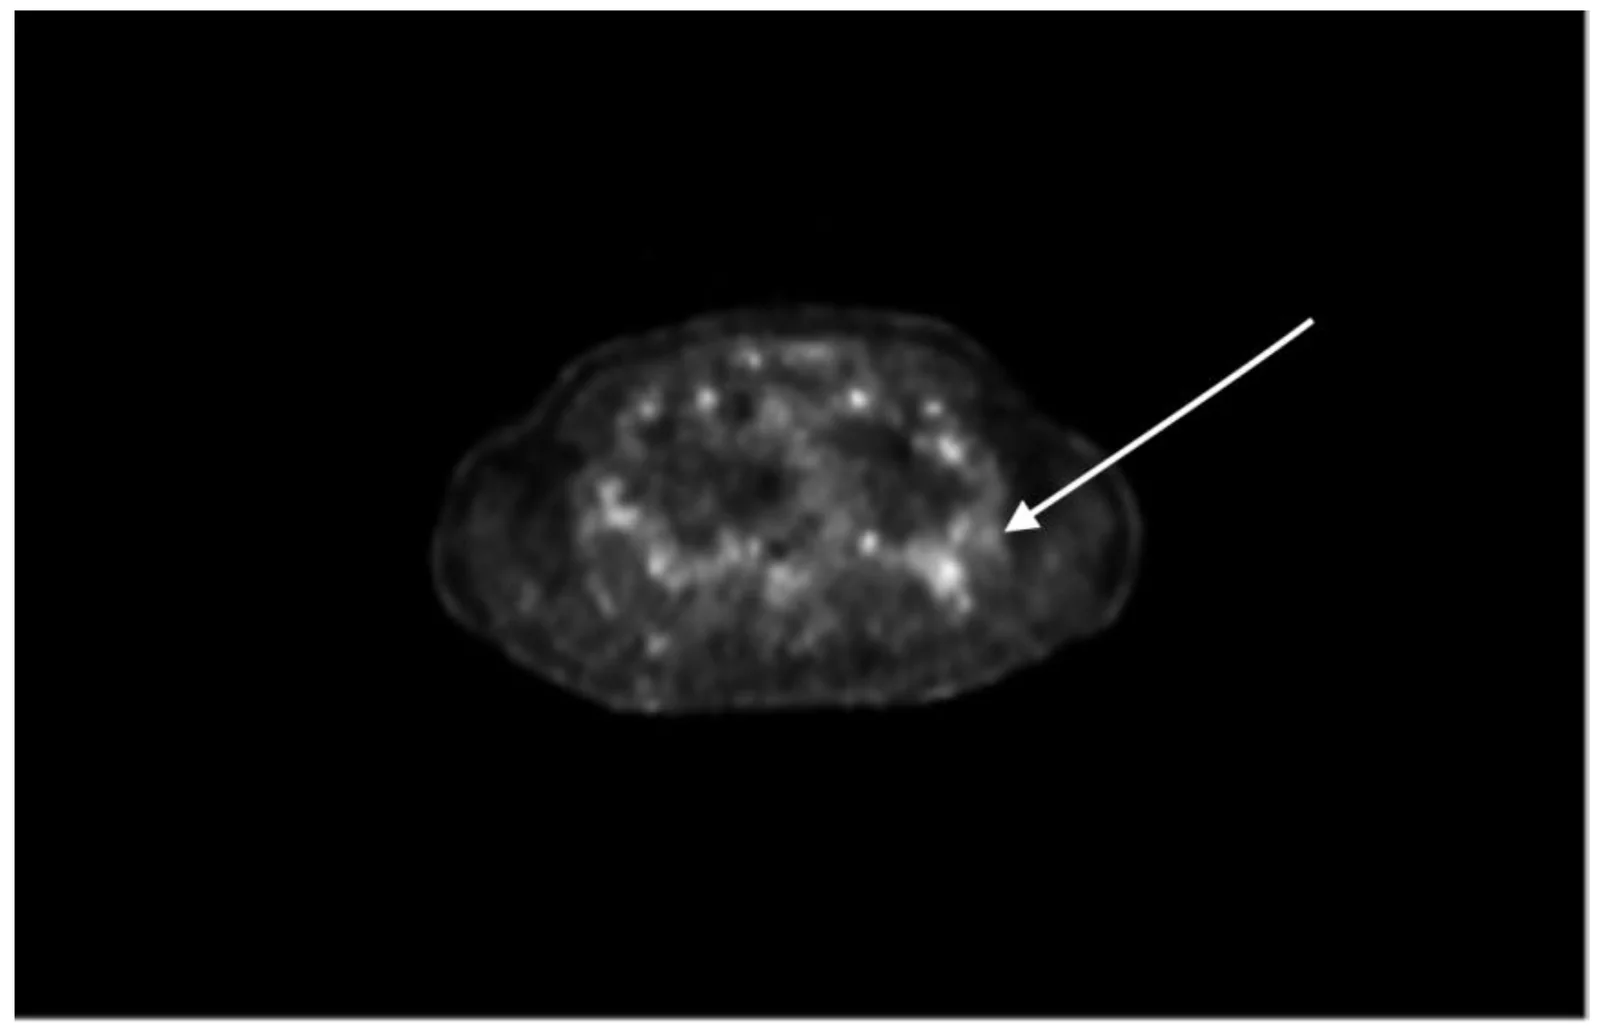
PET/CT imaging demonstrating a hypermetabolic mass in the left lower lung lobe (arrow), consistent with the suspected primary tumor.
